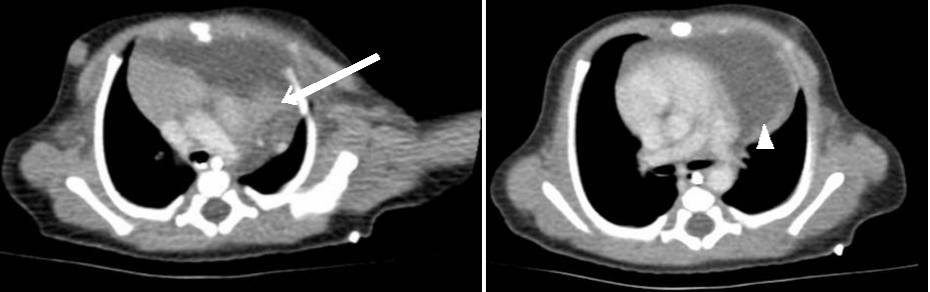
Contrast-enhanced computed tomography scan. Superiorly, this revealed a mixed attenuation, mainly cystic mass with a solid component within its left lateral aspect (arrow). More inferiorly, the lesion was of a more fluid density (arrowhead). These characteristics suggested a teratoma or congenital cystic adenomatoid malformation rather than cystic hygroma as the working diagnosis.
